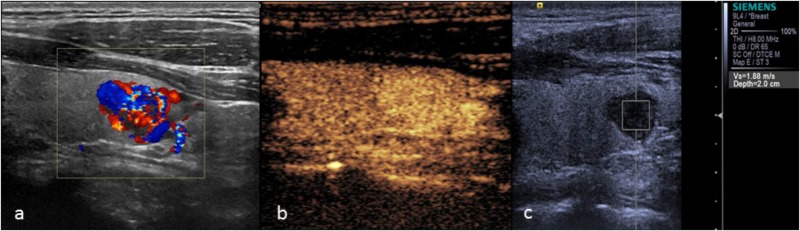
Thyroid follicular carcinoma. (a) Color Doppler ultrasound showing rich blood flow in the nodule. (b) CEUS showing heterogeneous homogeneous high enhancement. (c) The SWV value was 1.88 m/s.
